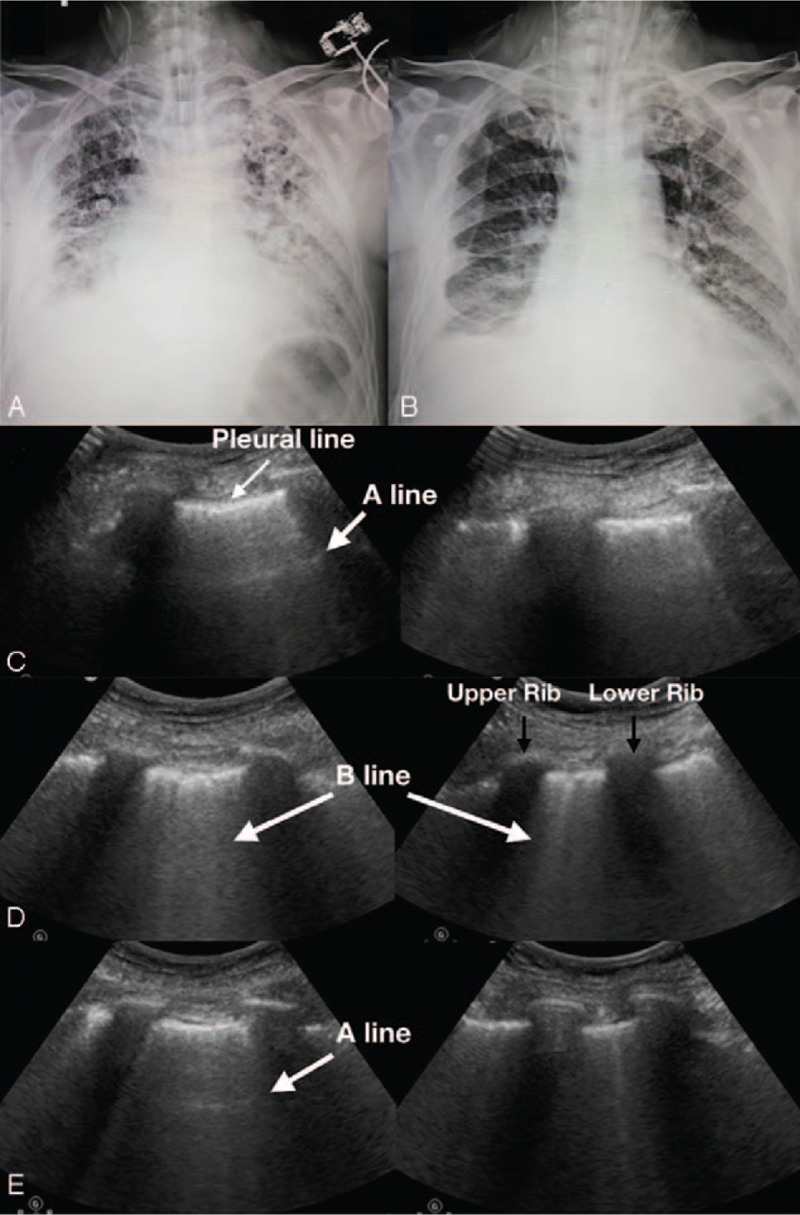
Imaging studies of chest x-ray and lung ultrasound. Chest x-ray showed bilateral diffuse and heterogeneous opacities on the first day of ICU admission (Panel A). Opacities diminished markedly within 1 week (Panel B) with bilateral A-line on the anterior chest (Panel C, left panel) and multiple B-line on the posterolateral chest wall (Panel C, right panel) evaluated by lung ultrasound examination. One day later, examination showed a marked increase of B lines bilaterally and a disappearance of A lines on the anterior chest (Panel D, left panel: upper point, right panel: lower point). After 24 hours of treatment, a repeated lung ultrasound revealed a marked decreased of B lines and A lines reappeared on the anterior chest. (Panel E, left panel: upper point, right panel: lower point). (The A line: the horizontal lines arising from the pleural line are separated by regular intervals that are equal to the distance between the skin and the pleural line. The A-line indicates air. The B line: multiple vertical comet-tail artifacts arising from the pleural line are spreading to the edge of the screen without fading and moving with lung sliding. It reflects the coexistence of elements with a major acoustic impedance gradient, such as fluid and air. Several B lines indicate increasing pulmonary fluid.). ICU = intensive care unit.
